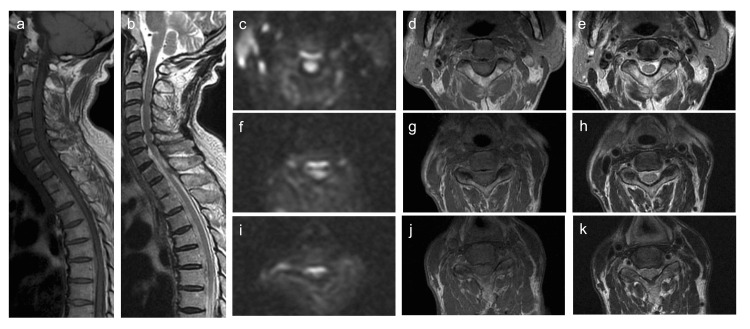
Spine magnetic resonance imaging findings on day 2 a: Sagittal T1-weighted image; b: Sagittal T2-weighted image; c, f, i: Axial diffusion-weighted images (DWI; b=650 s/mm2); d, g, j: Axial T1-weighted images; e, h, k: Axial T2-weighted images; c-e: At the C2 level; f-h: At the C5 level; i-k: At the C7 level. The hematoma spread thinly to the level of the lower thoracic spinal cord, with improved compression of the cervical spinal cord at the C2 level. DWI more clearly demonstrates the hematoma ventral to the spinal cord than axial T1- and T2-weighted images.
